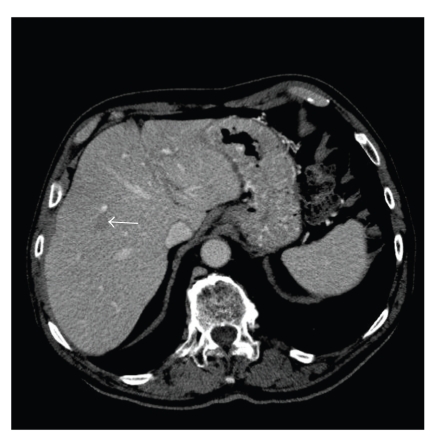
CT Scan showing segment 4b sclerosing haemangioma.
